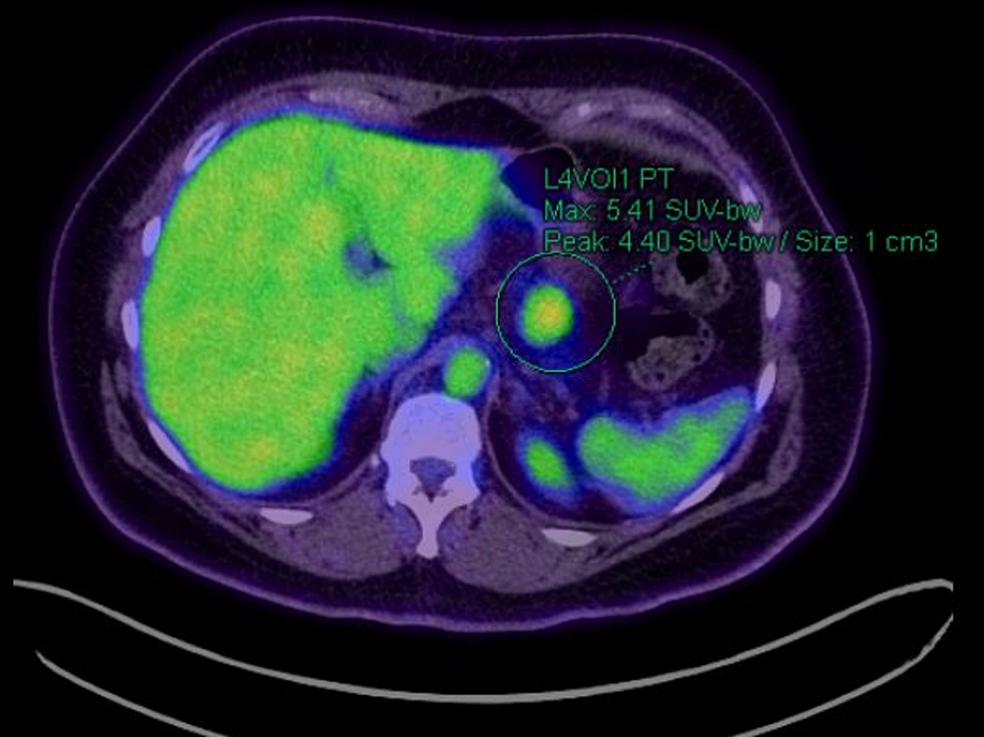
FDG-PET scan Green circle highlighting the pancreatic lesion being investigated. FDG-PET: fluorodeoxyglucose-positron emission tomography
